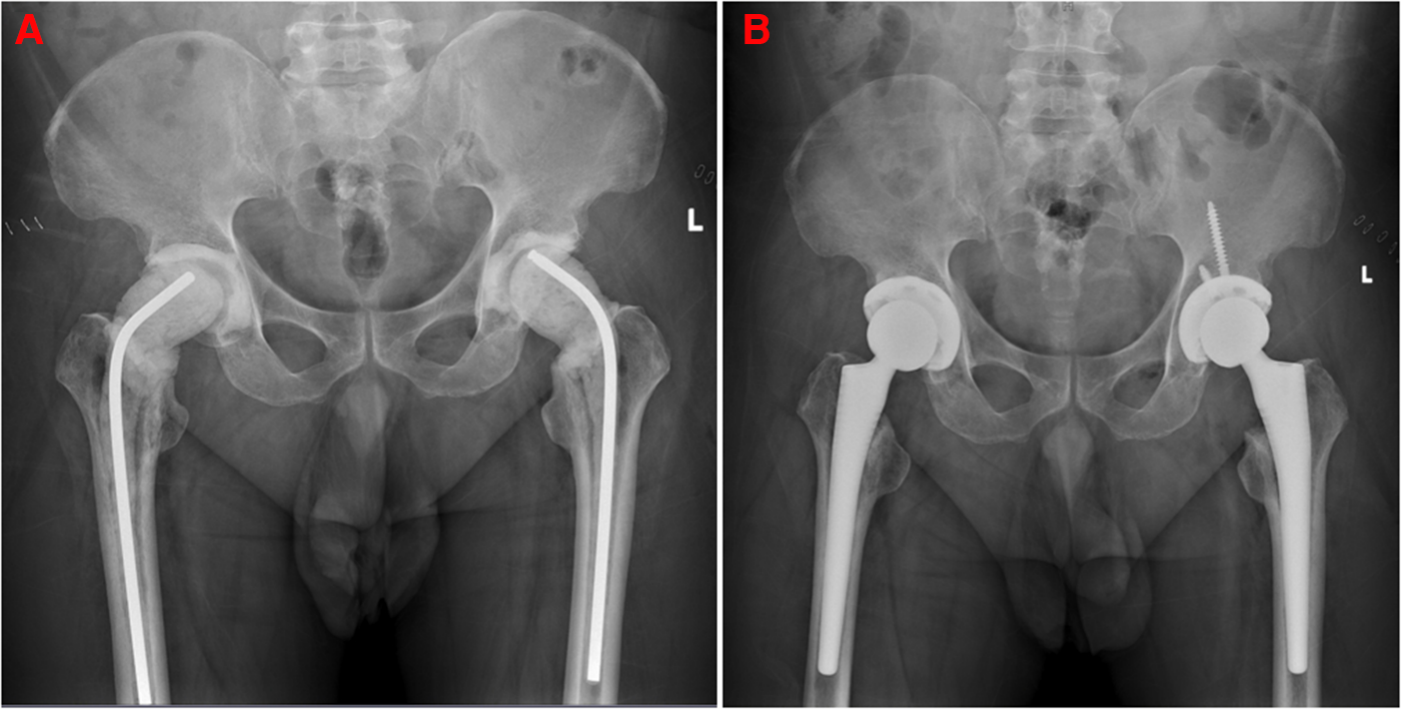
(A) Patient 5, anteroposterior radiograph of the hip taken after first-stage surgery. (B) shows an anteroposterior radiograph of the hip taken six months after stage 2 bilateral THA, indicating the prosthesis in place and good bone ingrowth without osteolysis.
